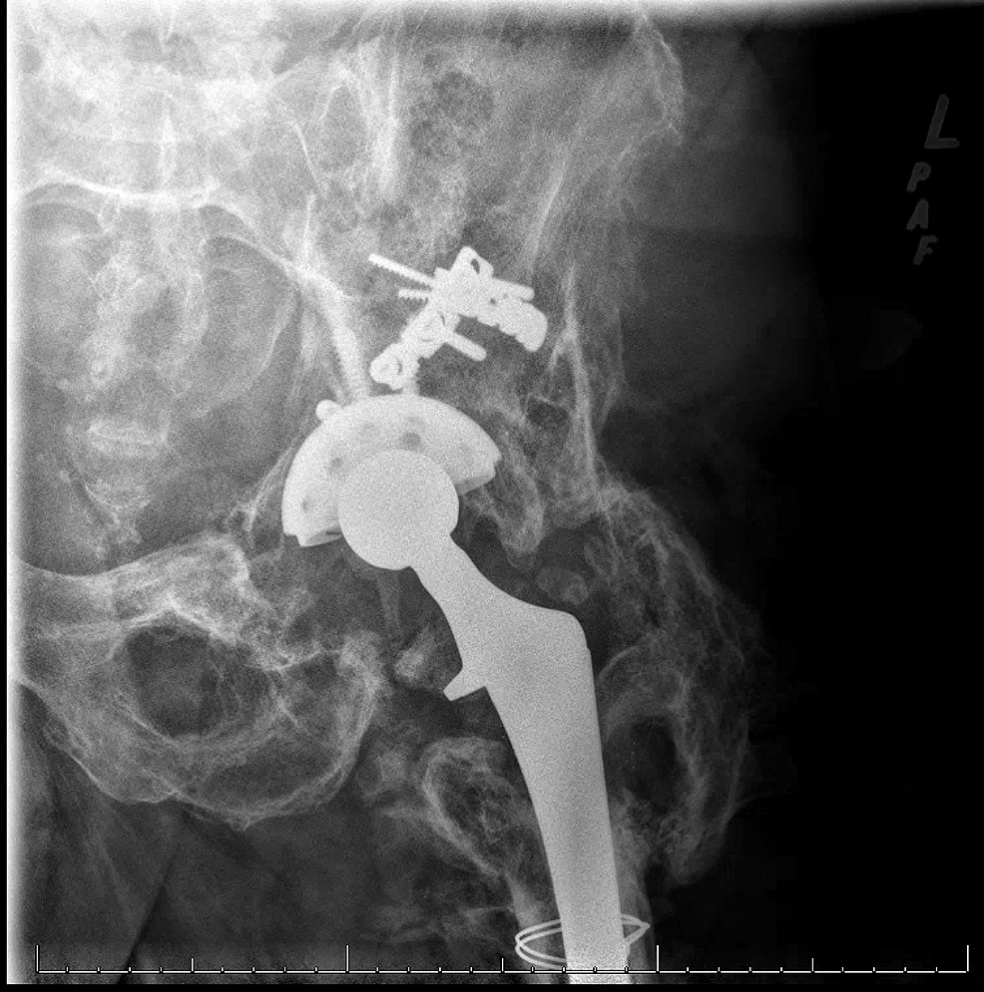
Postoperative radiographs. Postoperative anterior-posterior radiograph of the left hip demonstrating removal of broken acetabulum hardware and proximal cerclage wire with maintained left THA components in good alignment.
